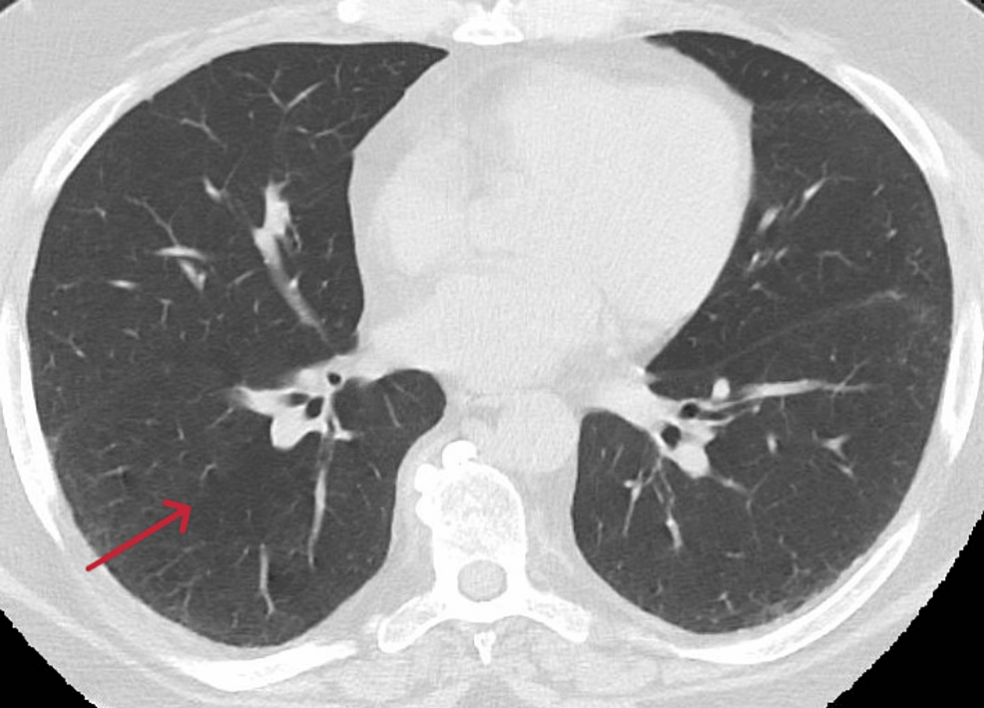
CT scan of the patient six months after treatment for ABPA: no pulmonary infiltrates CT: computed tomography, ABPA: allergic bronchopulmonary aspergillosis
